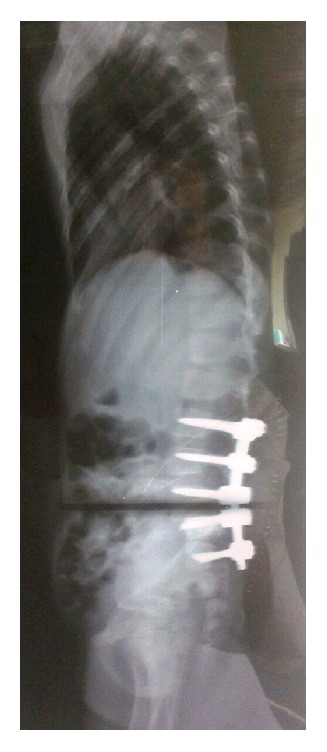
Postoperative standing lateral spine radiograph.
